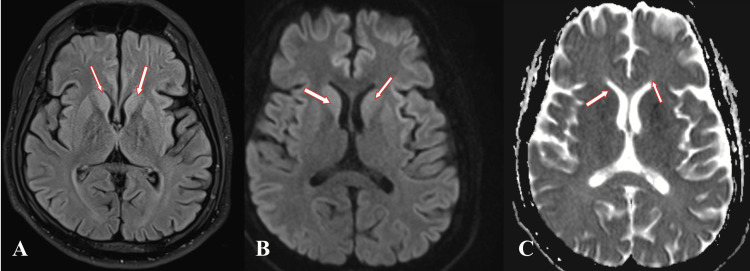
MRI brain with contrast of patient showing encephalitis changes. Hyperintense signals on FLAIR image (A) noted involving bilateral caudate nuclei, anterior aspect of bilateral putamen, and bilateral insular cortex (shown by red arrow), showing diffusion restriction on diffusion-weighted imaging sequence (B) with corresponding low apparent diffusion coefficient value (C). FLAIR: Fluid-Attenuated Inversion Recovery
